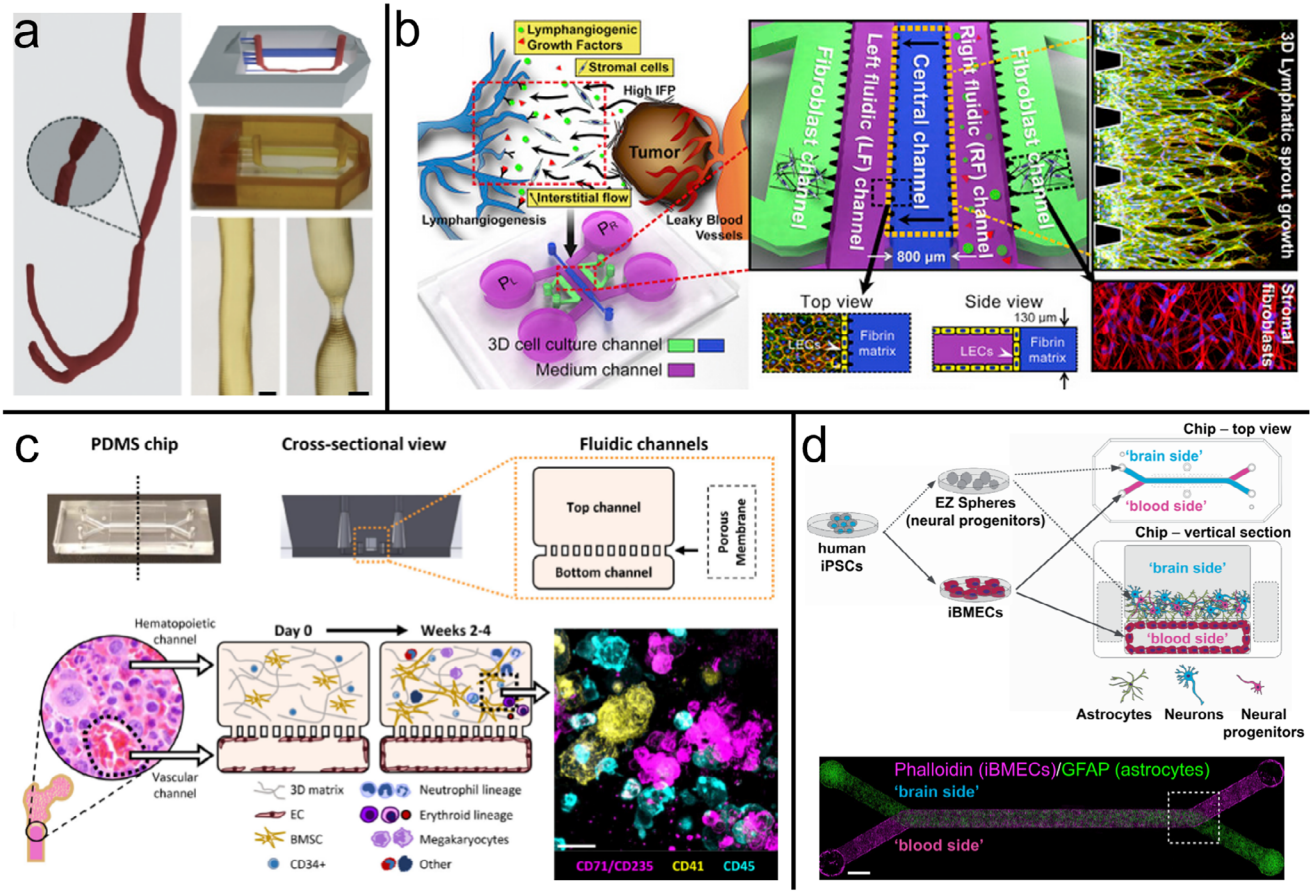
Endothelial barriers‐on‐chips. (a) Blood vessel OoC. Stenotic (and healthy) coronary artery segments were derived from computed tomography angiography and reproduced in an OoC using 3D printing to closely replicate physiological flow profiles for the resident endothelium. Adapted under the terms of the CC BY license [40]. Copyright 2017, Costa et al. (b) Lymphatic vessel OoC. The left and right fluidic channels (purple) allowed to adjust flow and biochemical factors for directional stimulation of lymphatic endothelium (LECs; yellow) seeded on a fibrin matrix (blue) to study lymphangiogenesis in this lateral/hydrogel OoC. Adapted with permission [42]. Copyright 2016, Elsevier. (c) Human bone marrow OoC. Incorporation of hematopoietic progenitors and bone marrow stromal cells (BMSC) in a 3D matrix above a bottom channel lined by endothelium (EC)s facilitated hematopoiesis in this commercial vertical/membrane chip. Adapted with permission [43]. Copyright 2020, Springer Nature. (d) Blood–brain barrier (BBB)‐OoC. Fully isogenic model with BBB iPSC‐endothelium (iBMECs; purple) and a heterogeneous population derived from iPSC‐neural progenitors inside a commercial vertical/membrane chip, employed to study two genetically‐driven disorders. Adapted with permission [44]. Copyright 2019, Elsevier. iPSC, induced pluripotent stem cell; OoC, organ‐on‐chip.
